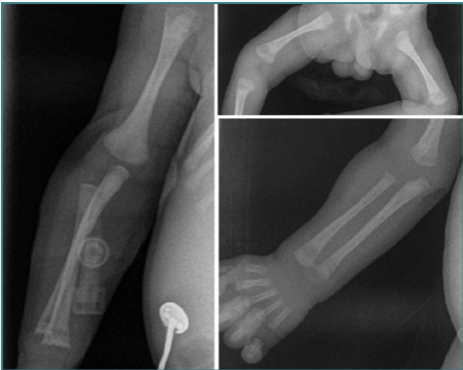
Radiographic findings of the long bones in a neonate
